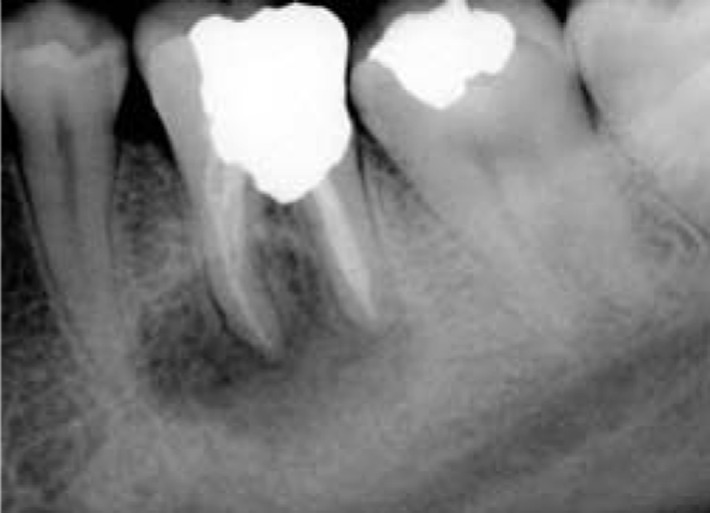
Preoperative radiograph of a mandibular first molar with a large apical lesion of the mesial root and furcal perforation repair subjected to IR.
